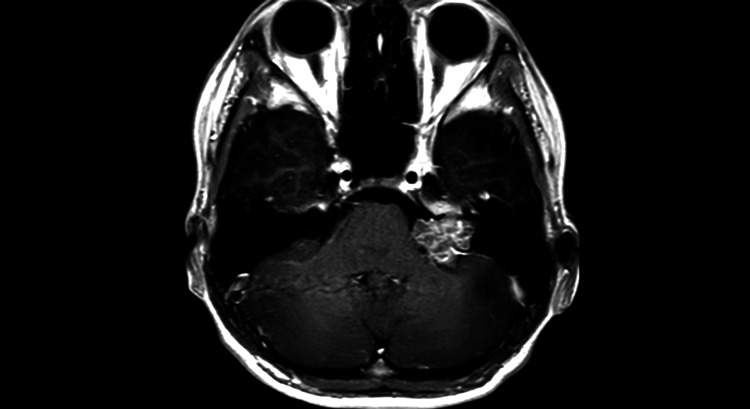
Preoperative MRI contrast-enhanced T1-weighted image A tumor shadow measuring 2.5 cm in the longest dimension was noted. It demonstrated heterogeneous contrast enhancement.
